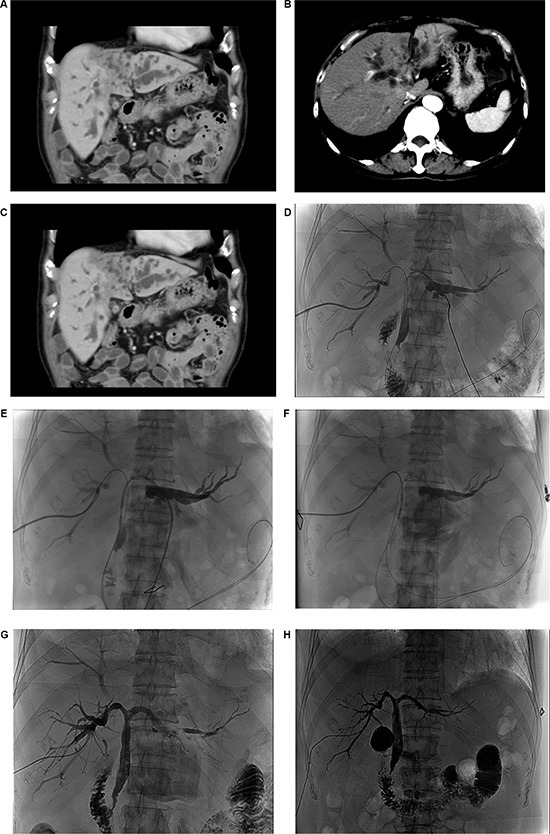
(A–C) Computed tomography scans show an inoperable Bismuth type IV hilar cholangiocarcinoma. (D) A percutaneous transhepatic cholangiography shows the stricture positioning. (E and F) Percutaneous radiofrequency ablation for the left and right hepatic duct. (G) Metal stent in position. (H) A cholangiogram showing stent patency four days after the RFA treatment.
